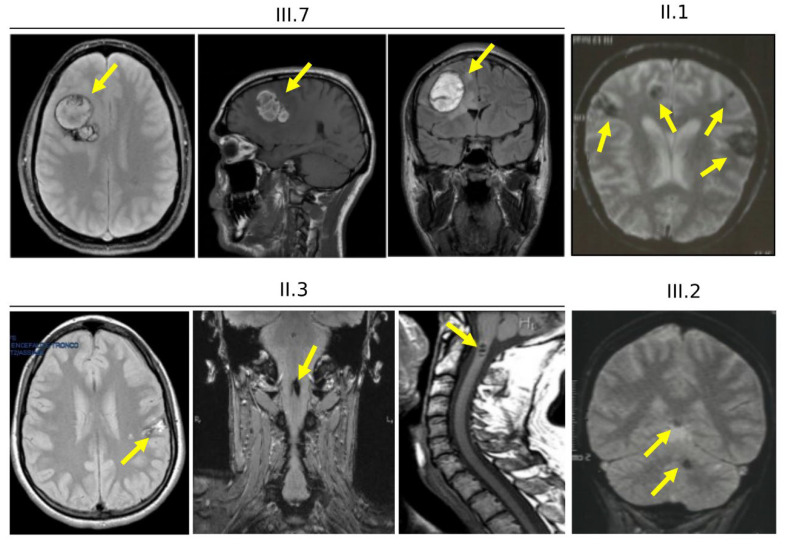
Brain and spinal cord magnetic resonance imaging of family 12. Representative brain and cervical spine MRI images of the proband (III.7) and three affected family members (II.1, II.3 and III.2) obtained with a 1.5T MRI scanner. Arrows indicate CCM lesions (also see Figure 1B and Supplementary Figure S1).
